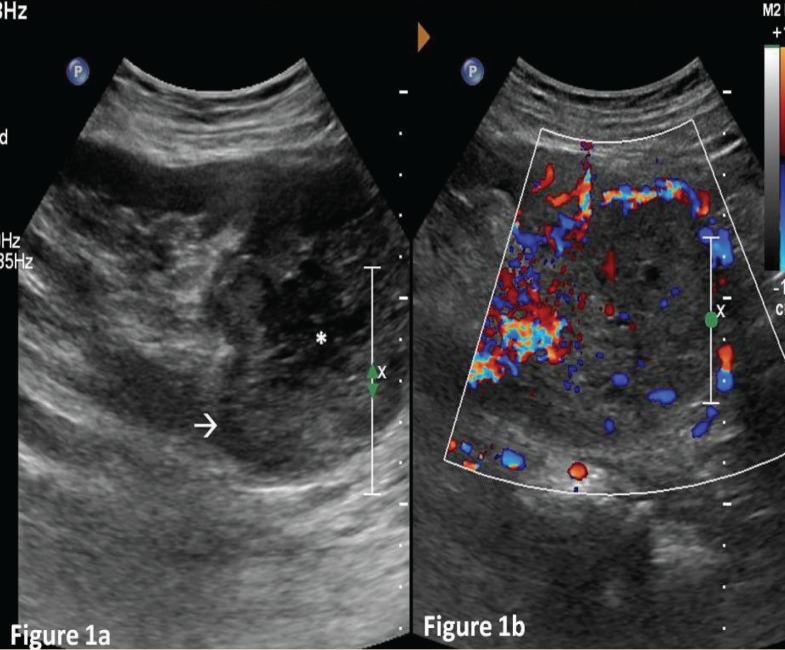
A 56-year-old man with renal cell carcinoma in renal allograft. Findings: Gray scale ultrasound image of renal allograft (a) heterogeneous mass lesion at the upper pole of renal allograft (white arrow) with internal hypoechoic region (asterisk) suggesting necrosis; (b) the mass lesion showed internal as well as peripheral vascularity on color doppler study (Technique: 2D-Ultrasound image scanned with Phillips IU-22 scanner and curvilinear C5-1 probe with frequency
